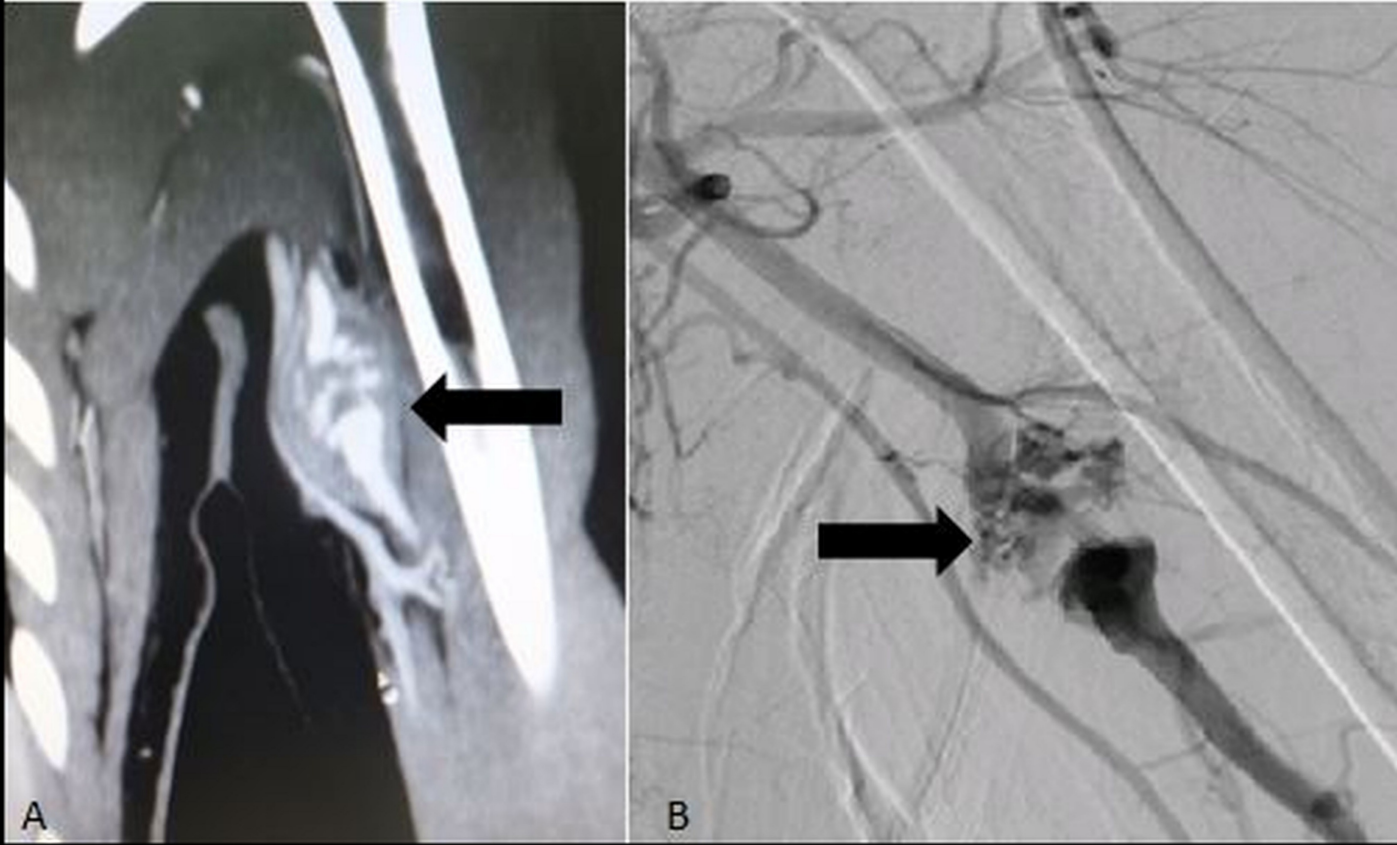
CT scan with contrast (A) and digital subtraction angiogram (B) of an 18-year-old patient showing multiple tiny outpouchings (Arrows) arising from the brachial artery
